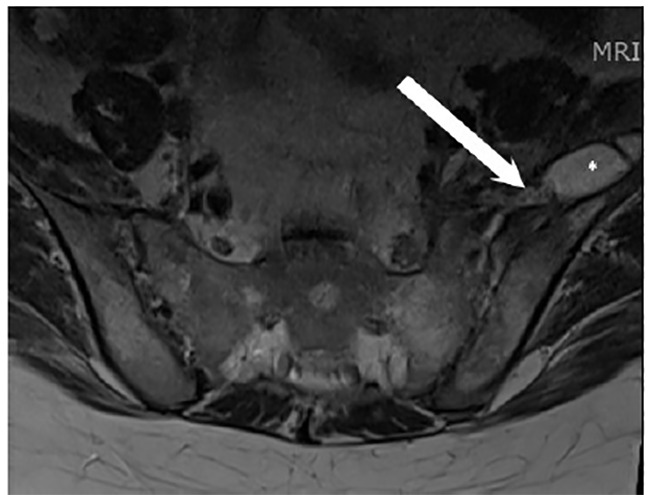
Axial T-2 weighted magnetic resonance imaging of lumbar spine after four weeks showing a recurrent abscess (*) measuring 3.2 x 1.3 centimeters. The lateral aspect of this collection extends beyond the field of view. The medial aspect of this collection appears continuous with the left sacroiliac joint (arrow), concerning for sacroilitis and possible involvement of the left iliac bone, although incompletely characterized on this study.
